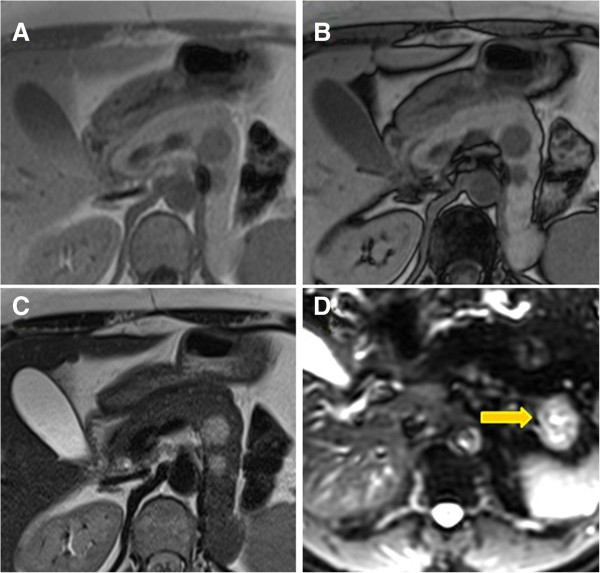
In-phase, out-of-phase and diffusion-weighted images. Lesions with signal intensity lower than normal pancreatic tissue on in-phase (A) and out-of-phase images (B). They showed hyperintense signal on T2-weighted (C) and diffusion-weighted sequences obtained at b values of 400 (D).
